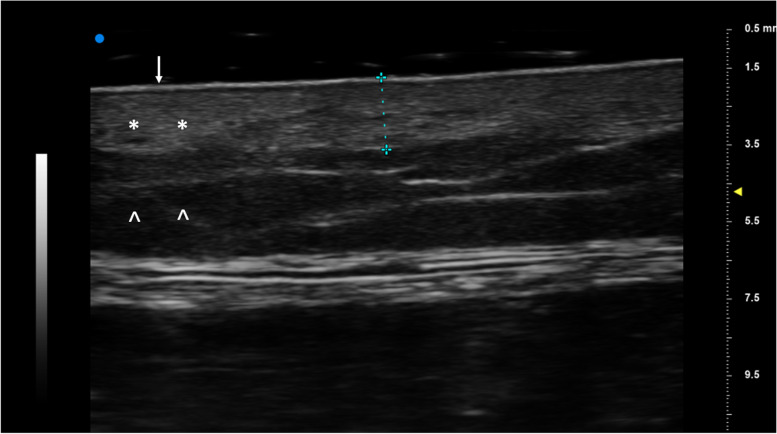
High frequency skin ultrasound image. High frequency ultrasound image of the pretibial soft tissues show the echogenic epidermis (arrow), heterogeneous and less echogenic dermis (*) and the hypoechoic hypodermis (^). (+ - - - +) Represents study measurement
